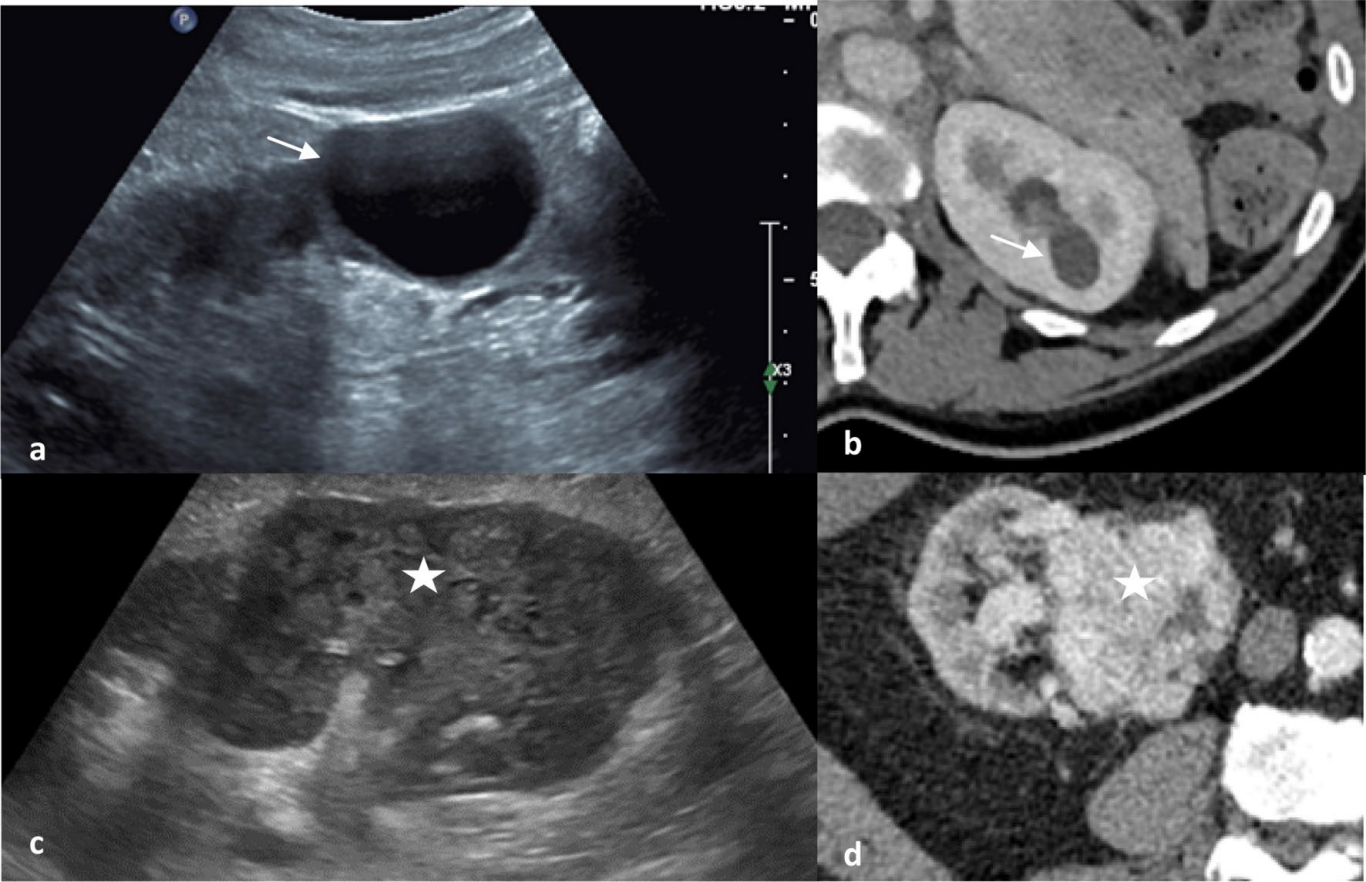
Ultrasound (a) and CT (b) appearances of a simple cyst with a thin imperceptible wall and posterior acoustic enhancement and no internal echoes or enhancement (Bosniak I). Contrast this to the ultrasound (c) and CT (d) features of a clear cell renal cell carcinoma with ill-defined borders and solid mixed echogenicity replacing renal parenchyma and avid contrast enhancement with areas of low attenuation tumoral necrosis on CT
